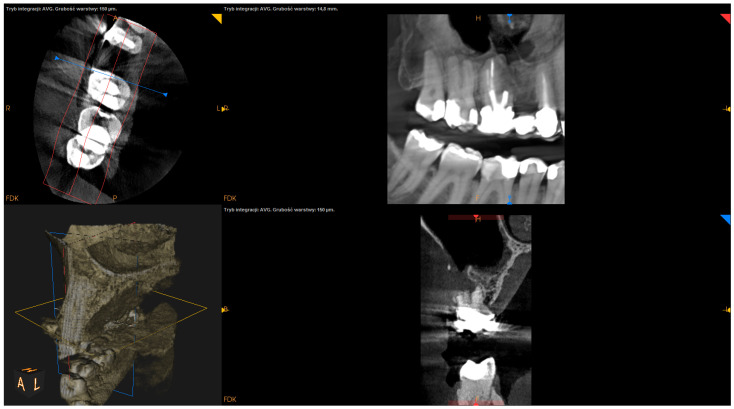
CBCT image (CS 8100 3D Carestream Dental^ⓡ^) example of unsatisfactory healing based on PENN 3D criteria. Non-English annotations present software technicalities (AVG integration mode and layer thickness).
